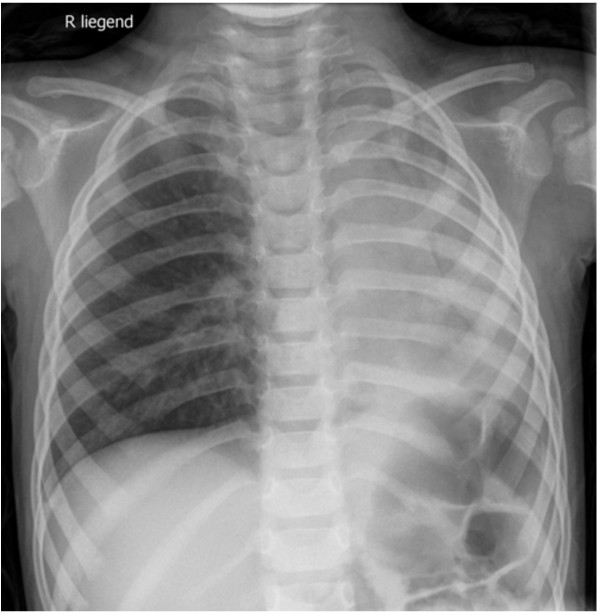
Chest X-ray of case 1 taken on admission. Abrupt termination of left main stem air shadow and collapse of left lung suggest complete obstruction of left bronchial tree.
